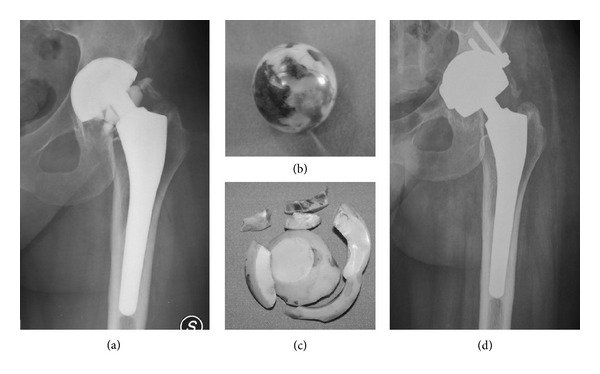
(a) Preoperative radiograph showing catastrophic failure of a ceramic liner. (b) The retrieved ceramic head grossly damaged because of contact with metal back. (c) The retrieved ceramic liner. (d) Postoperative radiograph after cup revision and bearing surfaces exchange to metal on metal coupling.
